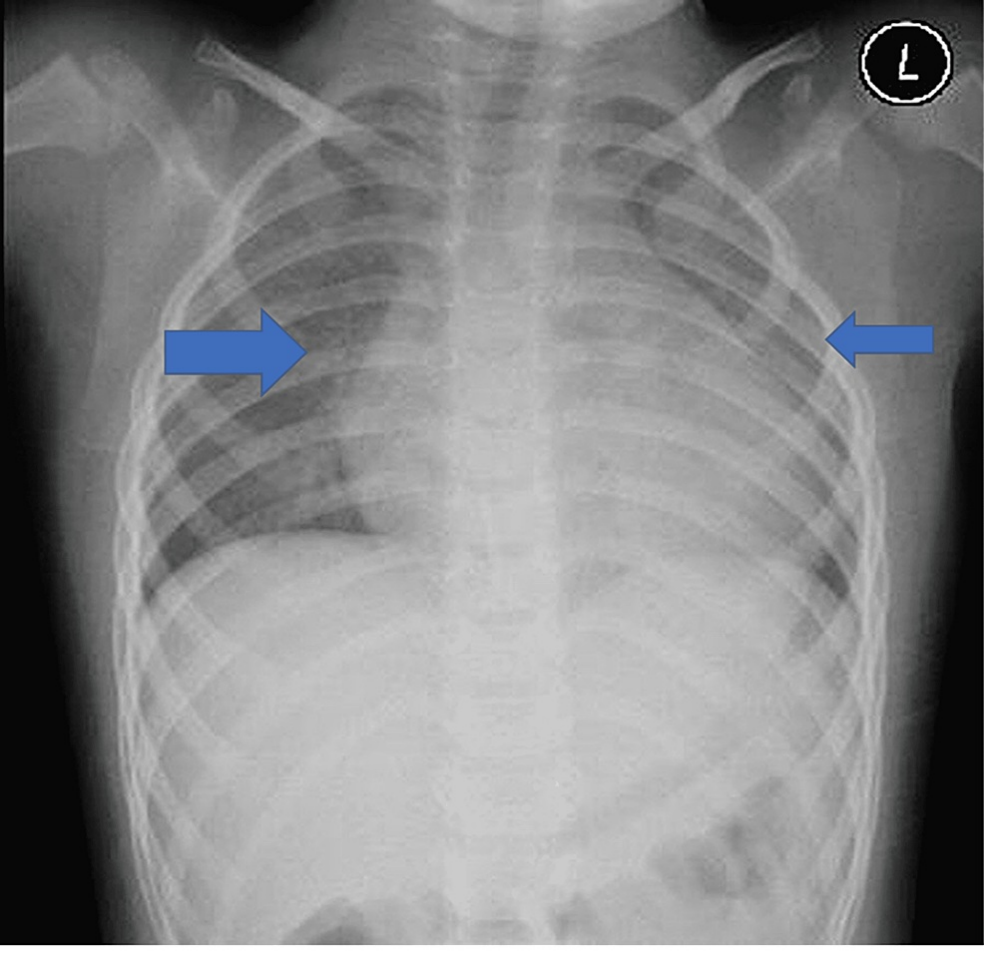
X-ray shows clear lungs fields apart from the bilateral peribronchial thickening (blue arrows).
